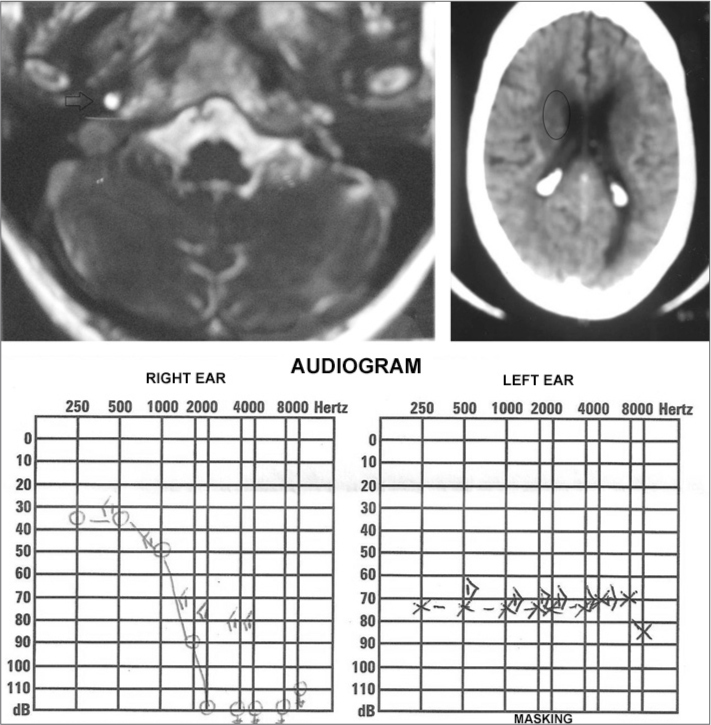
Complementary tests. Upper left-hand image: MRI showing an acoustic neuroma to the right (arrow); upper righthand image: skull CT showing a hypodense area near the right ventricle horn (circle); lower image: tonal audiometry showing bearing loss after a sudden hearing loss spell.
